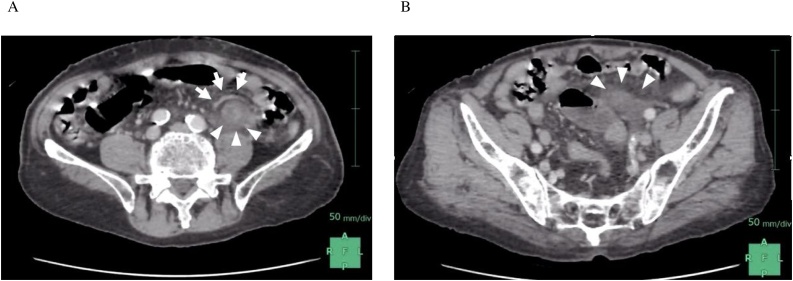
Contrast-enhanced CT on arrival. A. The herniated small intestine (arrows) on the dorsum of the vessel (arrowheads) in the sigmoid mesentery. B. Regional ascites around poor CT imaging of the herniated small intestine (arrows).
